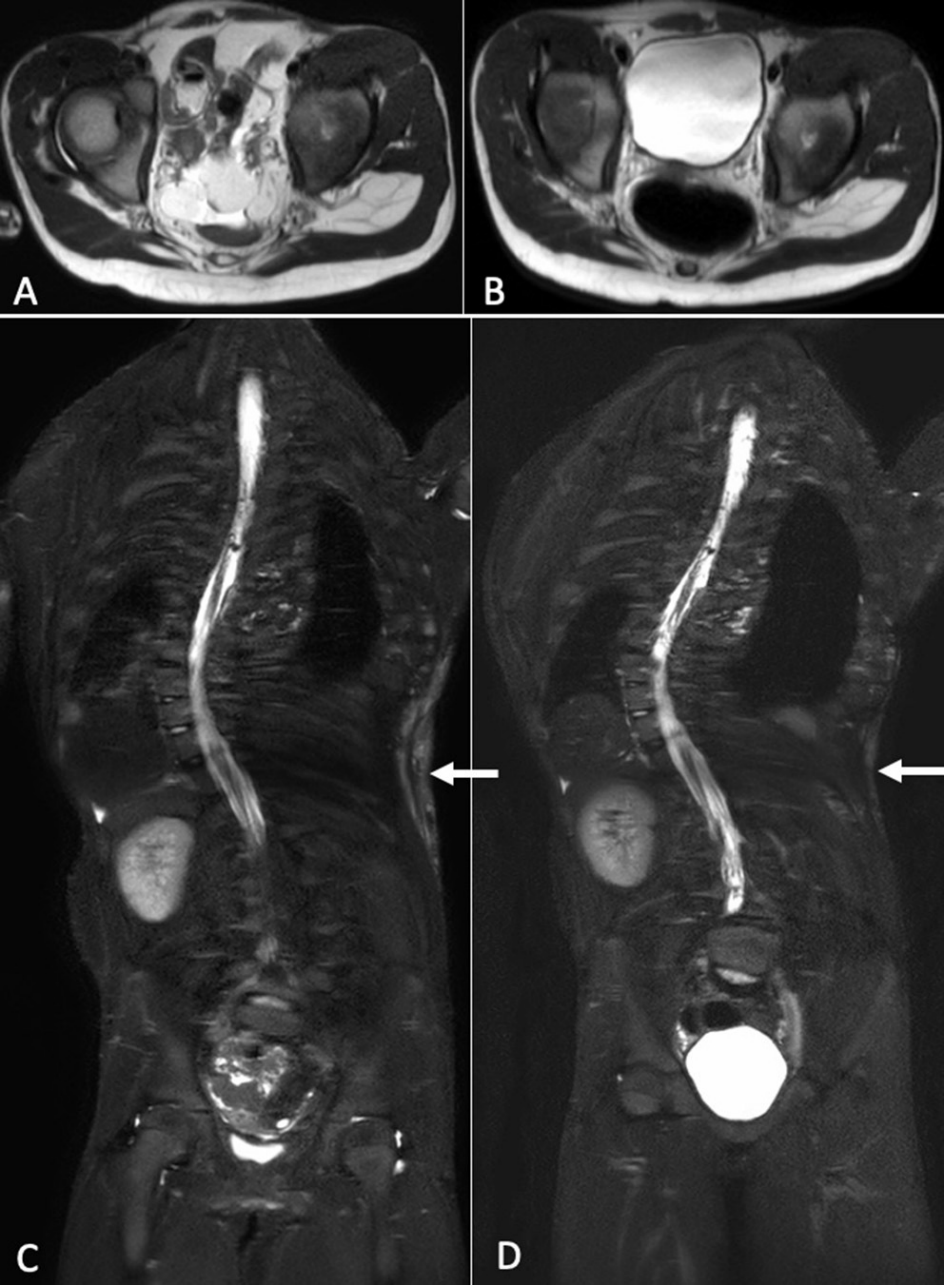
Axial T2-weighted MR images at baseline (A) and after about 6 months of therapy (B). A reduction of the soft tissues and visceral lipomatous overgrowth can be observed after treatment. Coronal STIR-weighted MR images show high-signal-intensity alteration in the soft tissues of the left flank consisting with low-flow vascular malformation, at baseline (C) and after therapy (D). After therapy, it was evident a reduction of the vascular malformation.
